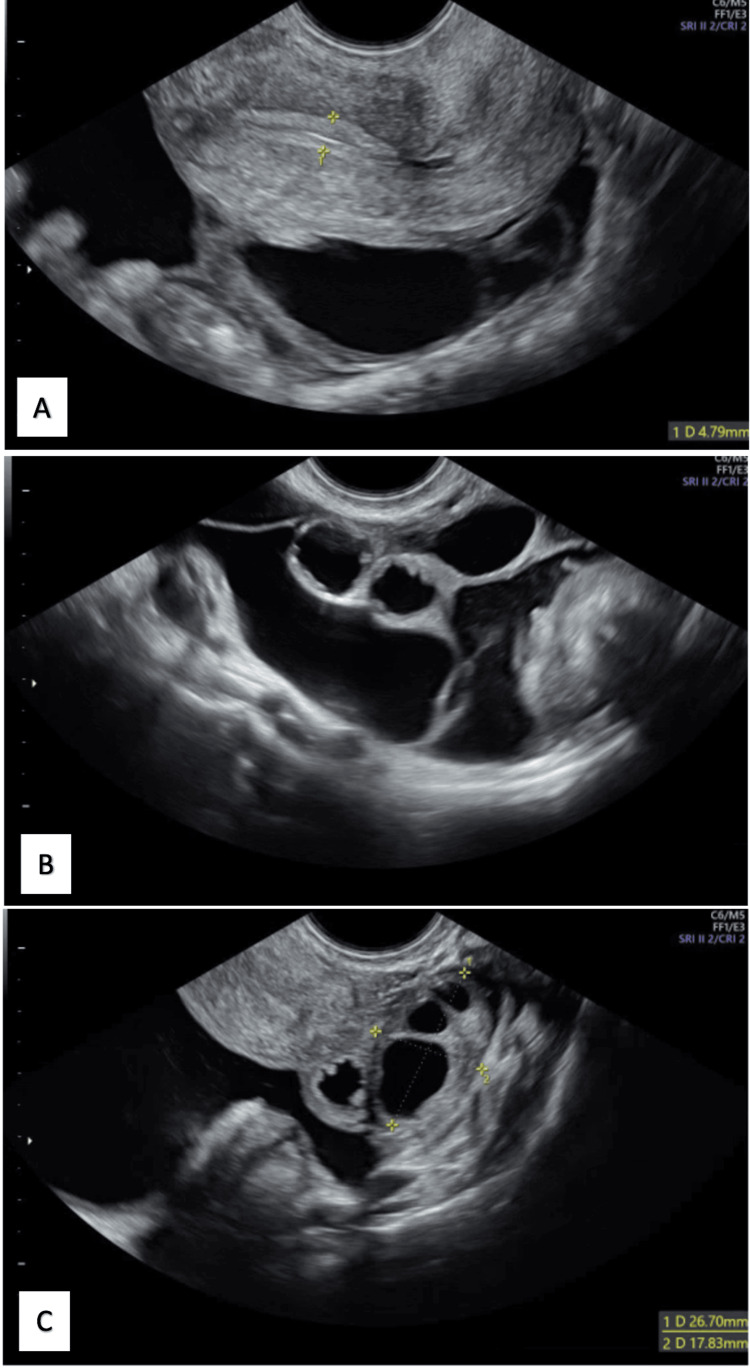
Transvaginal ultrasound evaluation before treatment (A) Uterus with a thin and regular endometrium, with apparently loculated anechogenic fluid, with thickened walls, in the pouch of Douglas. (B) Left adnexal area, with anechoic multiloculated cystic lesions, with hydrosalpinx, ascites and thickening of the peritoneum. (C) Right adnexal area, with an apparently normal ovary, is visualized.
